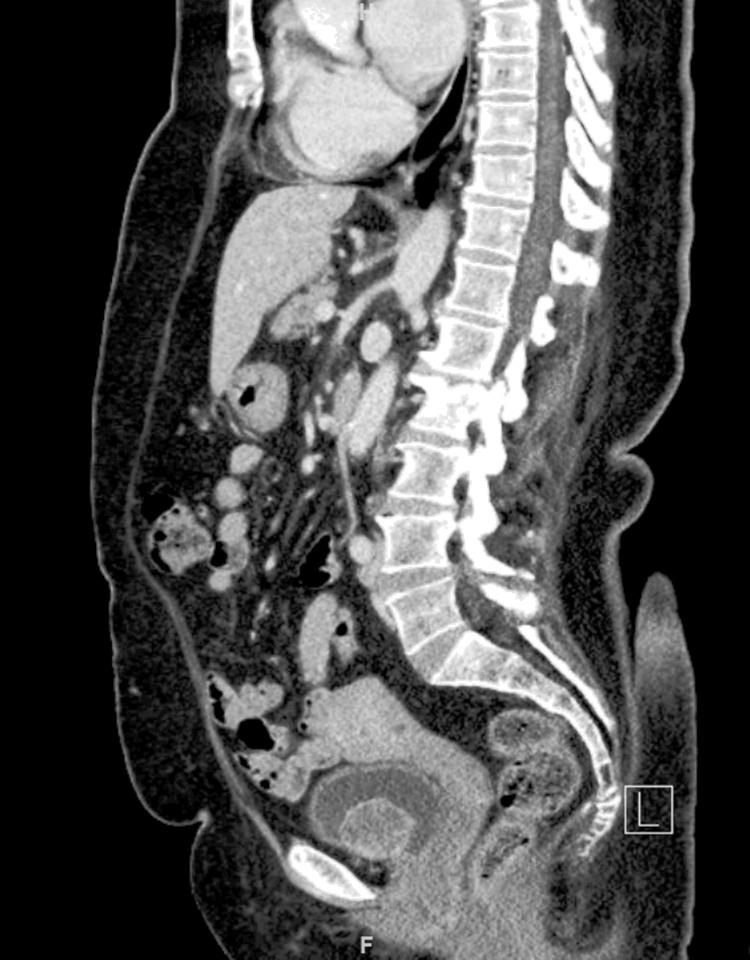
Sagittal reconstructed CT scan image showing bladder mass.
